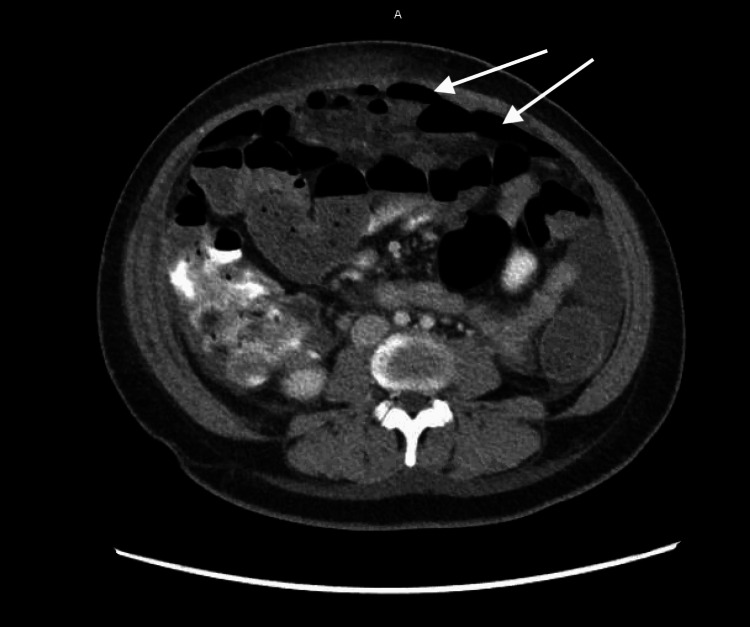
Contrast-enhanced CT of the mid-abdomen (axial). Arrows point to free intraperitoneal air consistent with pneumoperitoneum, most prominent in the anterior abdomen, concerning for a perforated viscus.
